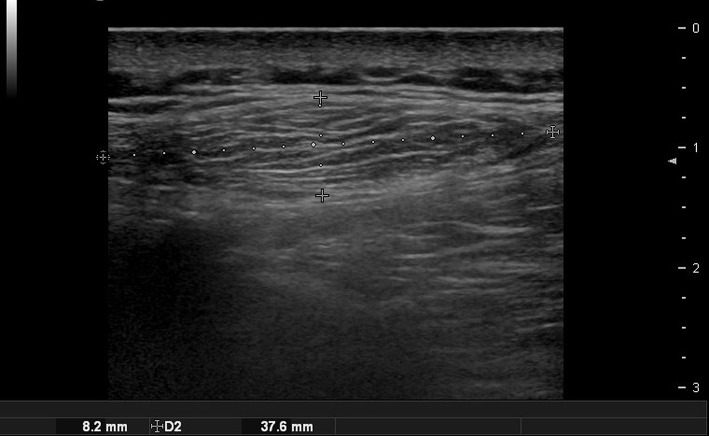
Ultrasound image of lipoma.
